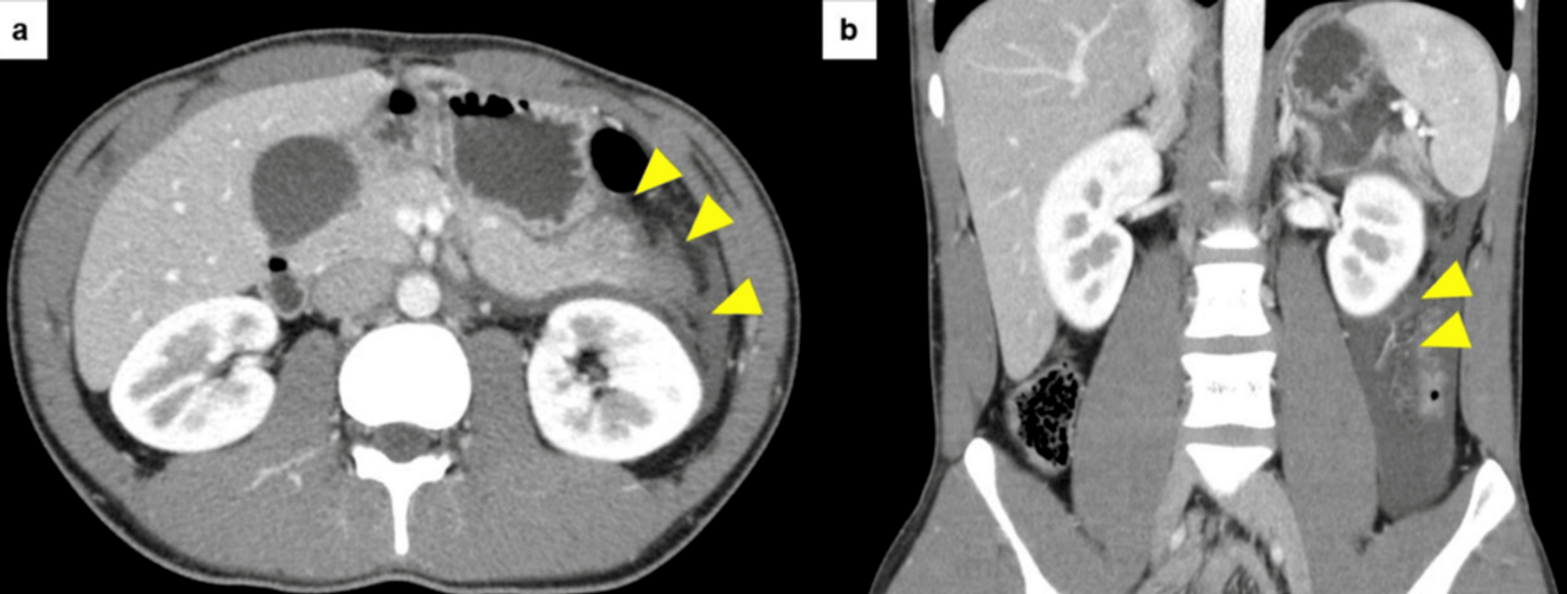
Abdominopelvic contrast-enhanced CT scan on admission. (a) Horizontal section image showing parenchymal enlargement and fluid retention in the pancreatic tail due to inflammation. (b) Coronal section image showing inflammation in the pancreatic tail extending to the lower pole of the kidney. Arrowheads indicate the spread of pancreatitis inflammation.
